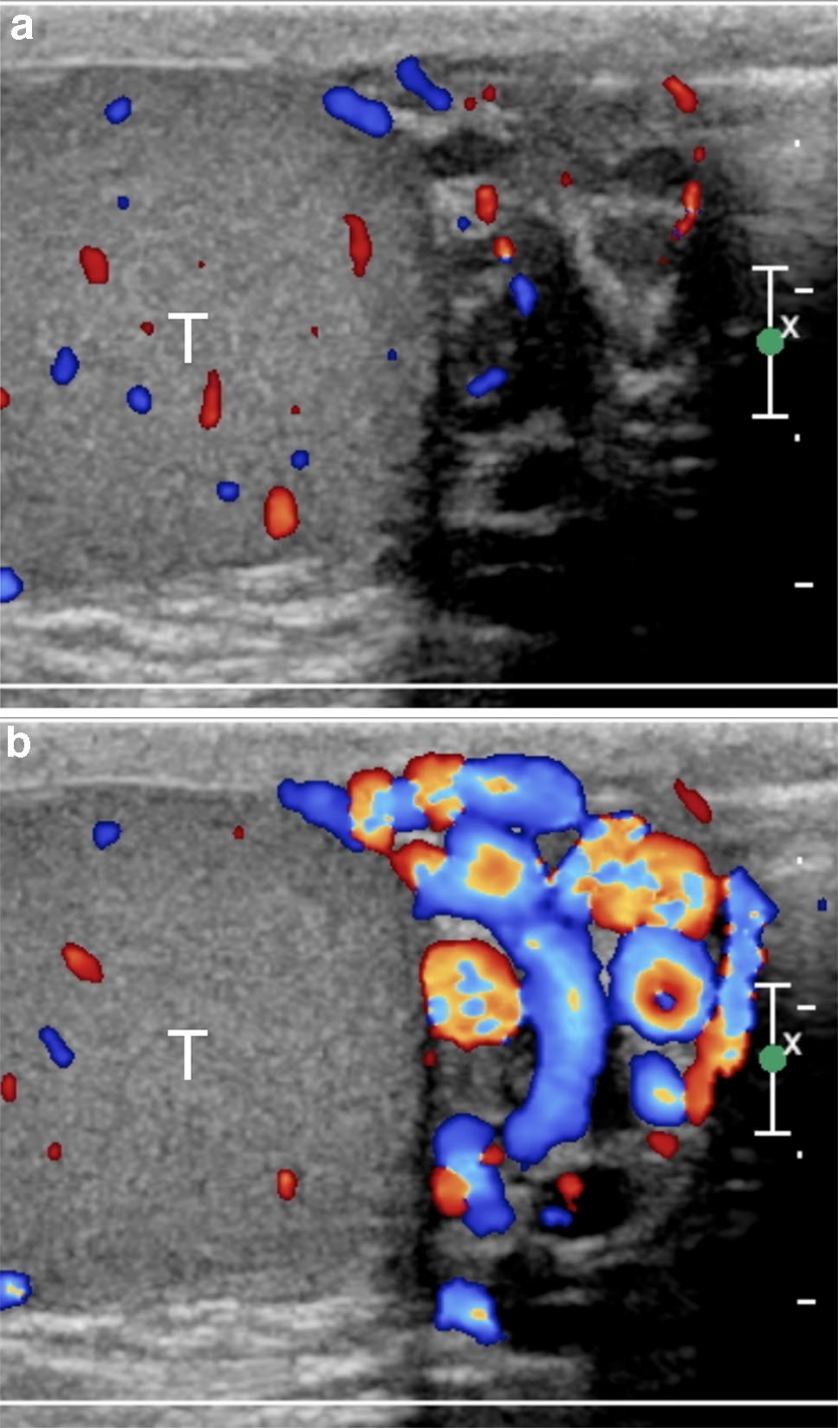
Sarteschi’s grade III varicocele. Colour Doppler images obtained at rest (a) and during Valsalva (b) showing dilated veins to the inferior pole of the testis (T) with reflux during Valsalva
